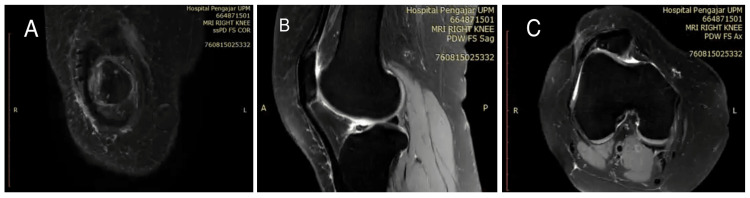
Images A to C showing MRI PDW-FS of right knee in coronal, sagittal, and axial cuts. MRI: Magnetic resonance imaging; PDW-FS: proton-density weighted in fat suppressed
